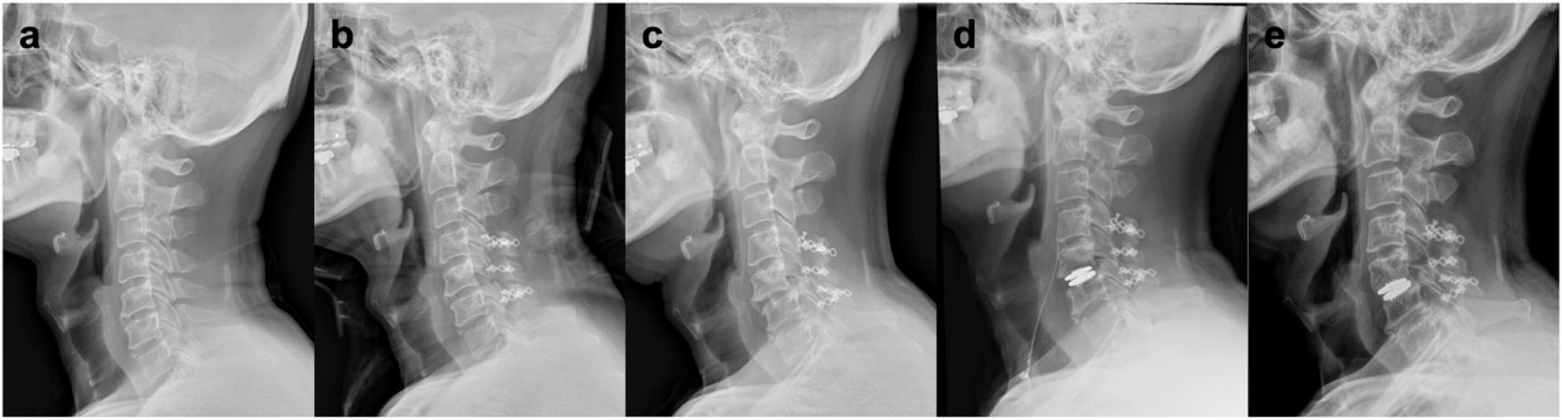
Sequential lateral cervical spine radiographs demonstrating postoperative evolution following staged resection of a C5–6 dumbbell meningioma. a Preoperative radiograph showing preserved cervical alignment. b Early postoperative image after the second-stage ventrolateral resection with right C5–6 facetectomy, demonstrating stable alignment without instrumentation. c Follow-up radiograph at 2.5 years showing progressive C5–6 spondylolisthesis, consistent with delayed instability after facetectomy. d Post–artificial disc replacement (ADR) radiograph demonstrating restoration of disc height and cervical alignment. e Follow-up radiograph at 3 years after ADR showing a stable implant
